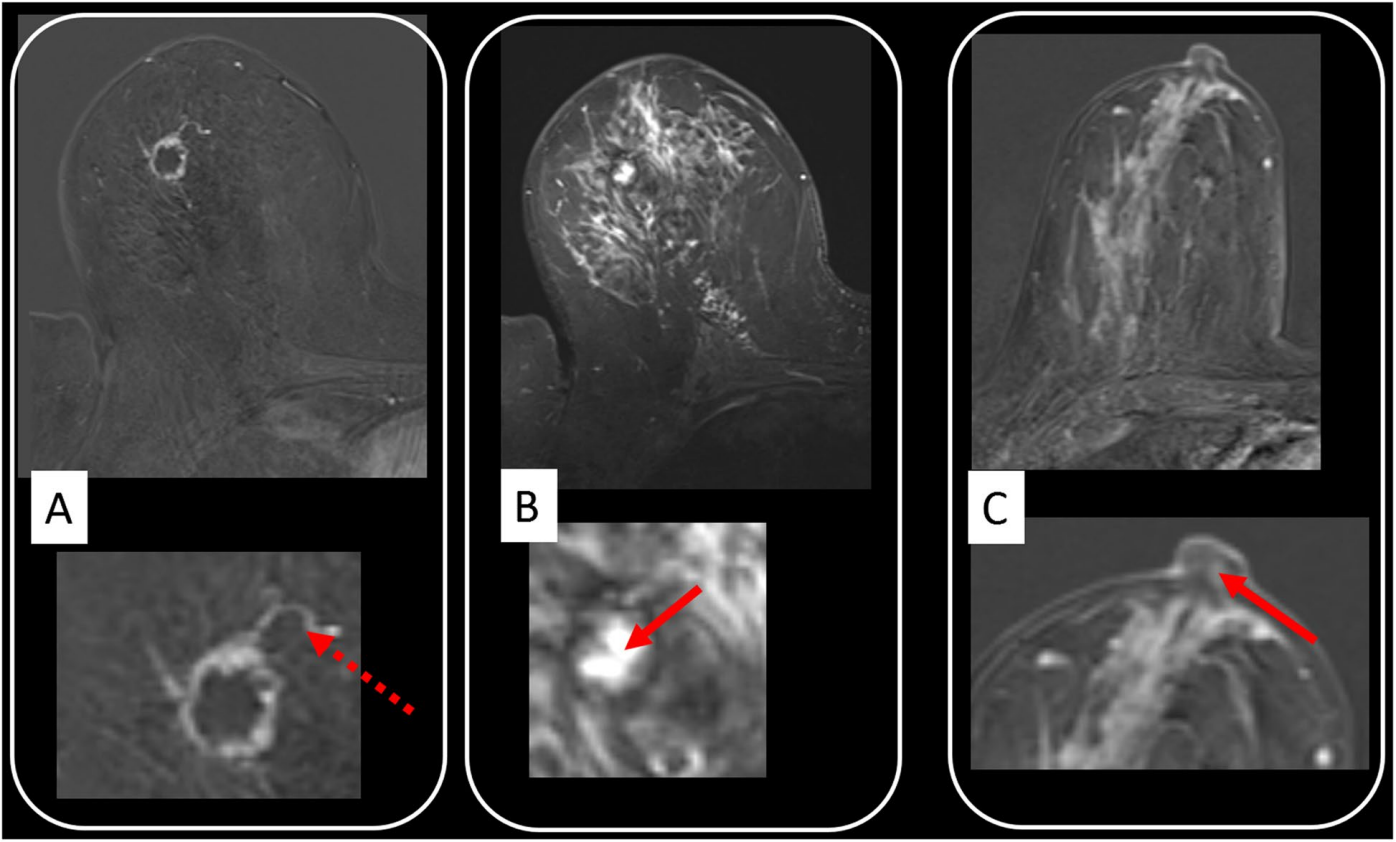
Selected semantic parameters with known biological correlates. They can be readily implemented into clinical practice. Rim enhancement (A) was among the first morphologic parameters reported in the literature and reflects an aggressive cancers phenotype. This is also suggested by the adjacent vessel sign (A, magnification: dotted red arrow) [50]. Rim enhancement is thought to reflect central hypovascularity due to connective tissue, fibrosis, and/or necrosis. Necrosis sign (B) specifically depicts central colliquative (liquid) necrosis (B, magnification: full red arrow), characterised by a high signal intensity on T2-weighted images within the centre of the tumour. Invasion of the cancer into the nipple areolar complex is related to poor outcome. The semantic criterion described as “destruction of nipple line” (C) is best depicted on DCE images (C, magnification: red arrow). Further details including diagnostic performance of semantic parameters are provided in Table 1
